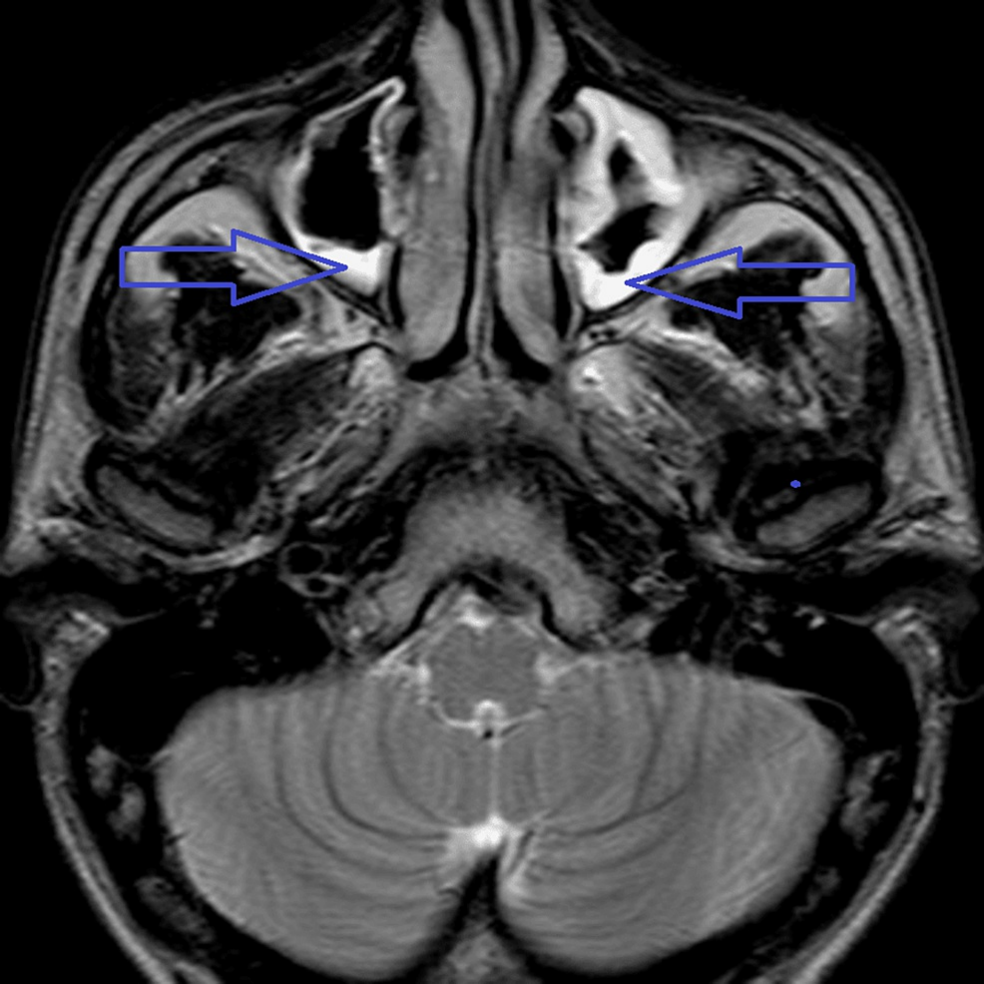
Axial T2-weighted image reveals mucosal thickening of both maxillary sinuses (arrows).
